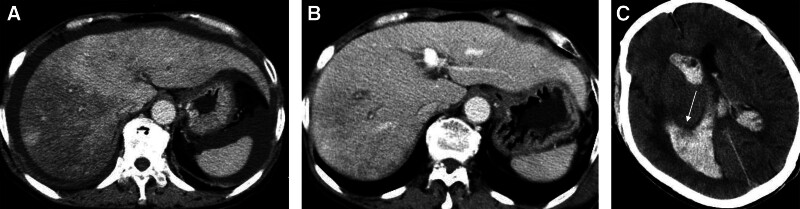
A 52-year-old woman with abdominal pain and distension after using a water-based decoction of Tusanqi for 20 days (A). After 20 days of treatment with low-molecular-weight heparin and warfarin, the liver’s density was uniform, the ascites remitted, and the hepatic veins were clearly visible (B). After she suddenly lost consciousness, computed tomography revealed an intraventricular hemorrhage (C, arrow).
